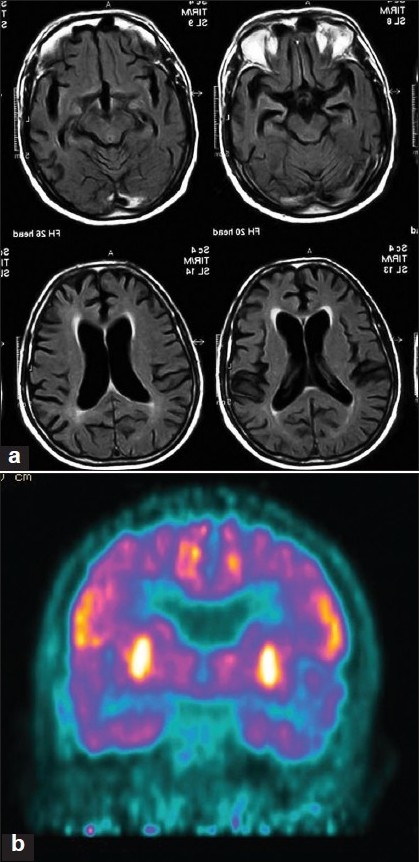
(a) MRI brain T1W axial image showing diffuse atrophy in a 58-year-old man with logopenic aphasia. (b) FDG PET-CT showing hypometabolism in bilateral temporal lobes
